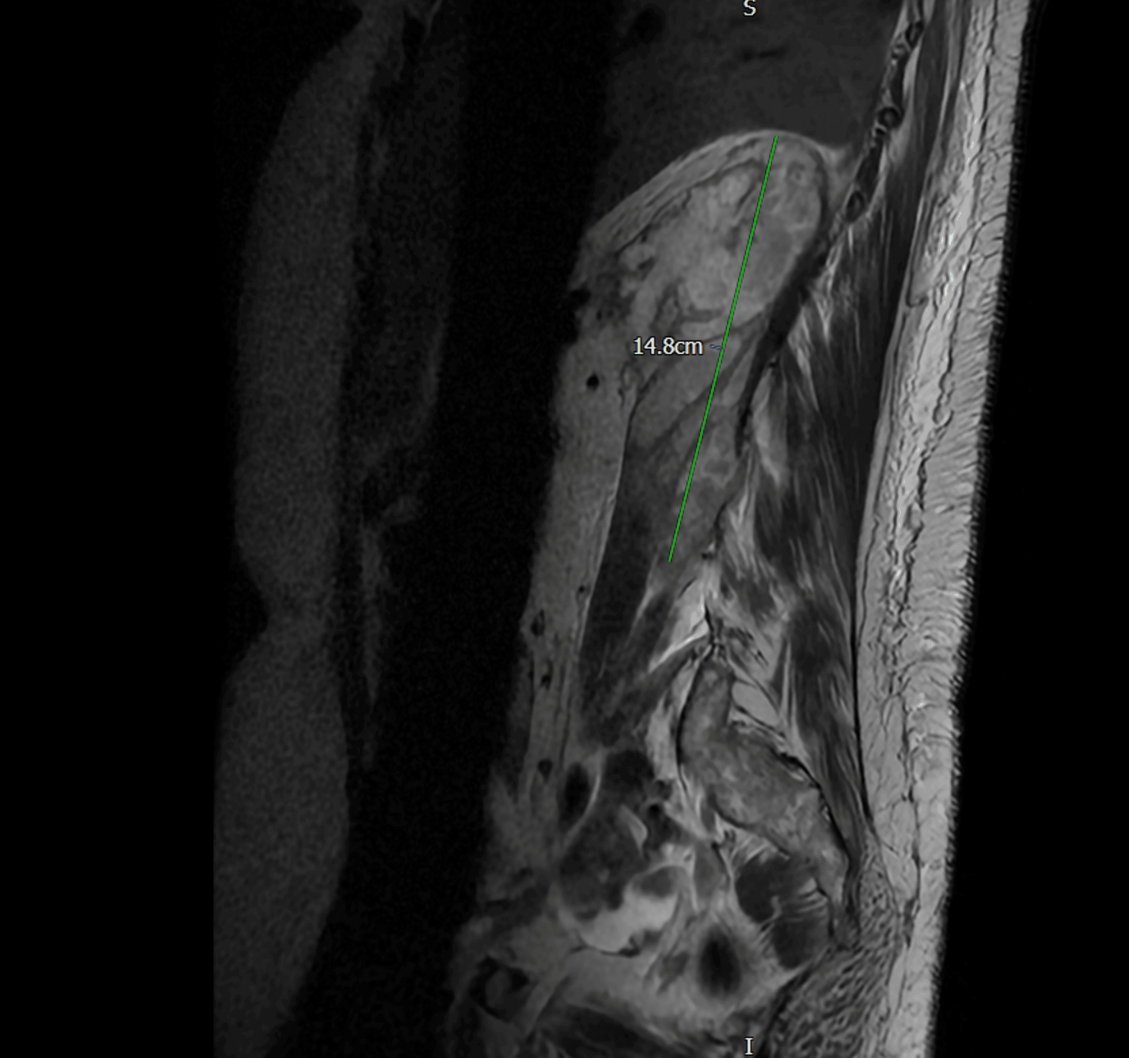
MRI whole spine done on day 2 shows large retroperitoneal collection in the posterior perinephric space and right iliopsoas muscle MRI: magnetic resonance imaging
